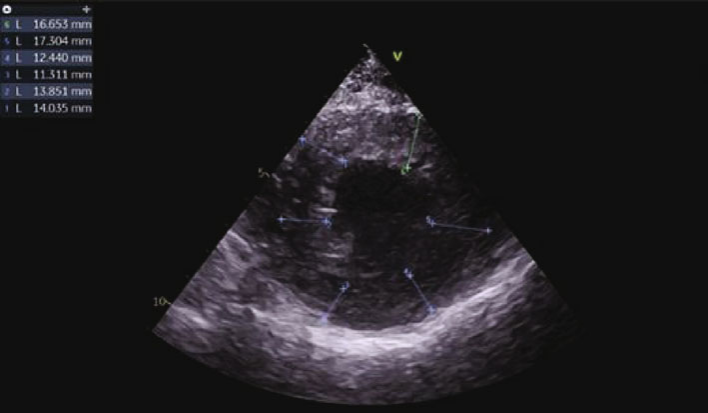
Echocardiography on reexamination showing little thickening of the left ventricular wall.
